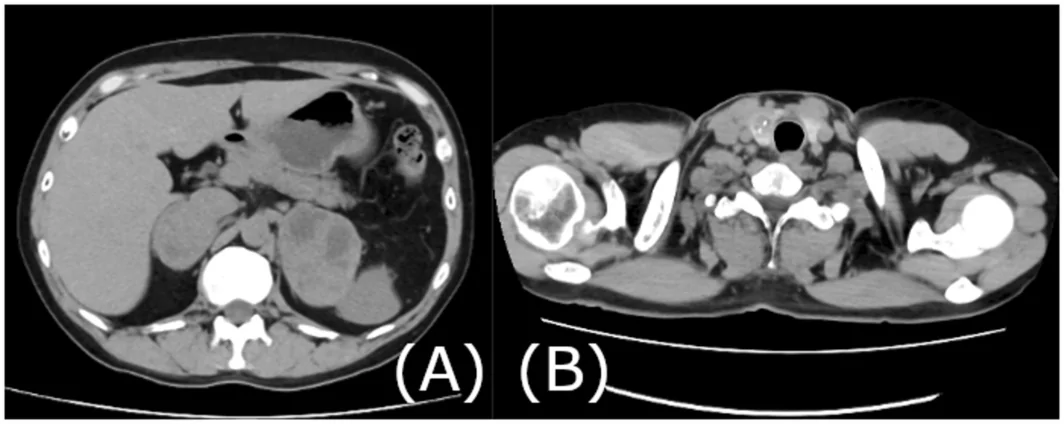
Computed tomography findings. (A) Plain computed tomography demonstrating bilateral adrenal tumors measuring 4.9 cm on the right and 7.7 cm on the left. (B) Plain computed tomography demonstrating a thyroid nodule posterior to the right thyroid lobe.
